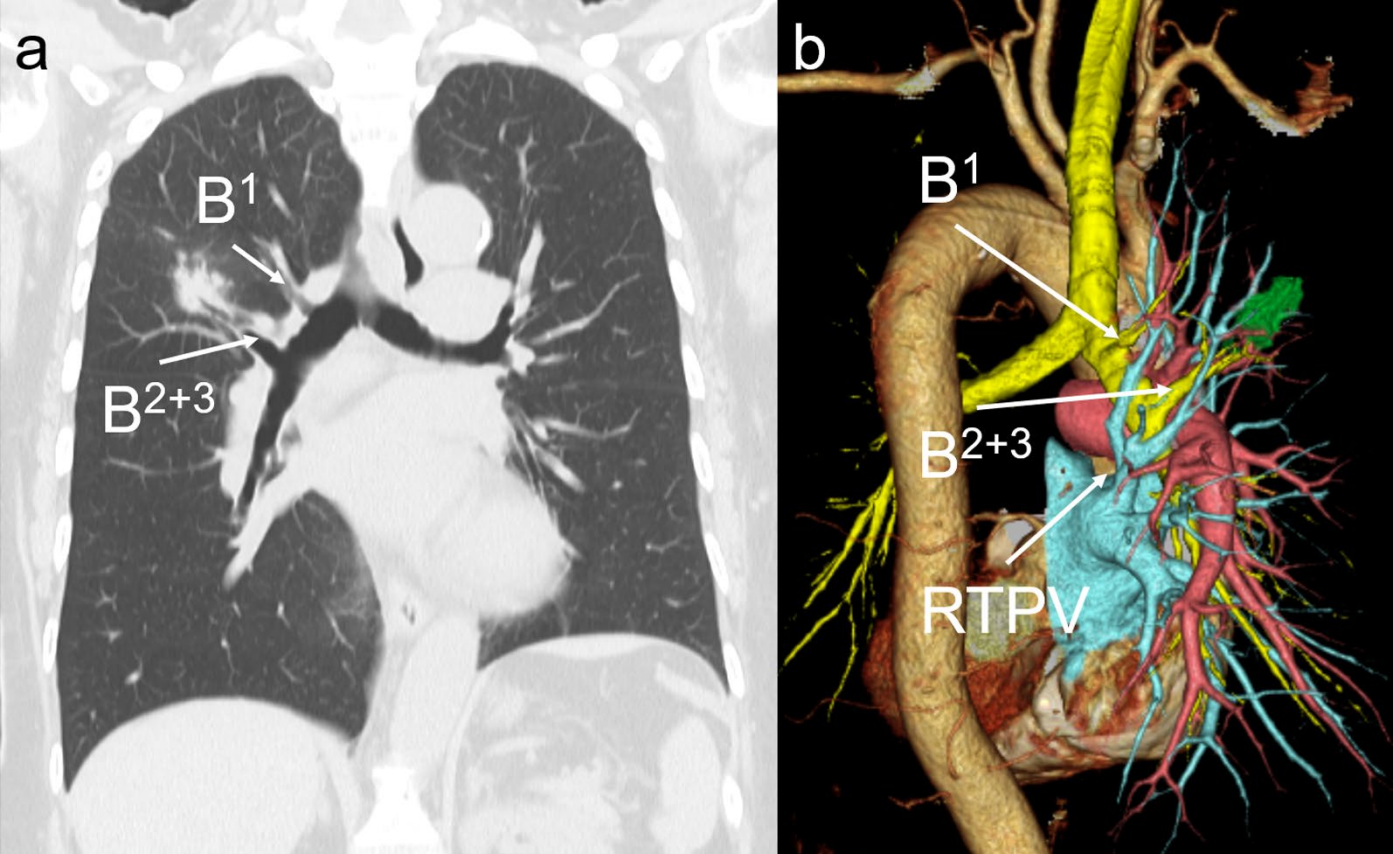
Preoperative findings of Patient 2. Chest computed tomography (CT) revealed: a a displaced right apical bronchus (B^1^) and common stem of the dorsal and ventral bronchus (B^2+3^) arising from the right main bronchus (RMB) independently, and b a right top pulmonary vein (RTPV)
